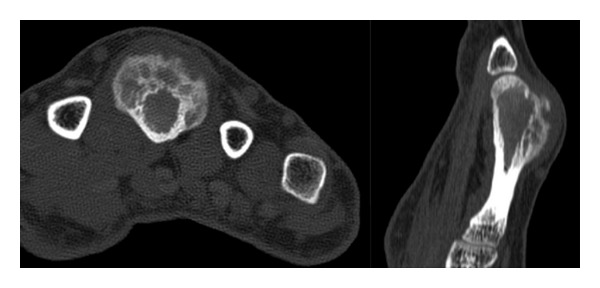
CT axial and sagittal images of the third metacarpal demonstrating a small focal area of continuity between the medullary cavity of the lesion and that of the underlying bone.
